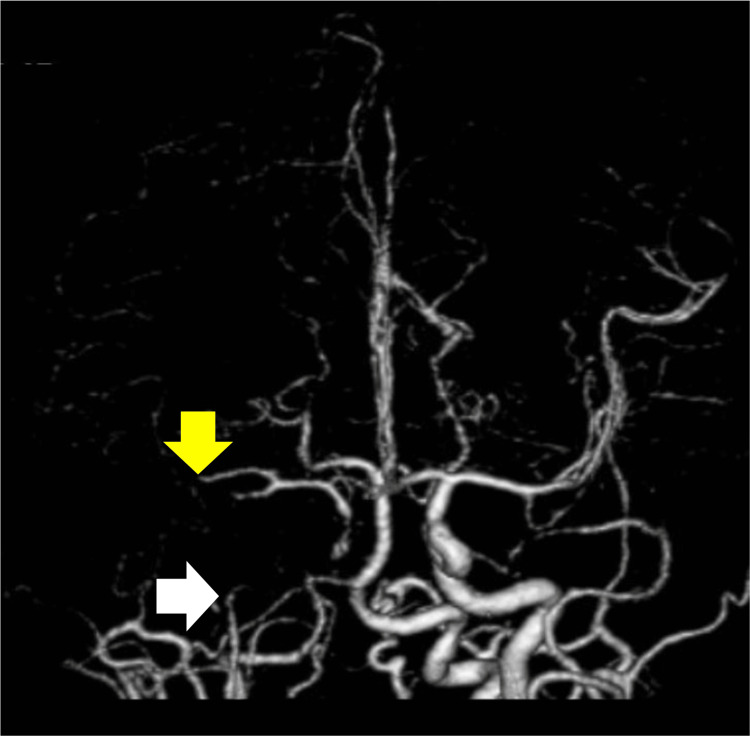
Brain three-dimensional CT angiography on day 0 (frontal view). On day 0, a three-dimensional CT angiogram showed a stenosis-like appearance in the right intracranial internal carotid artery (white arrow) and middle cerebral artery (yellow arrow).
